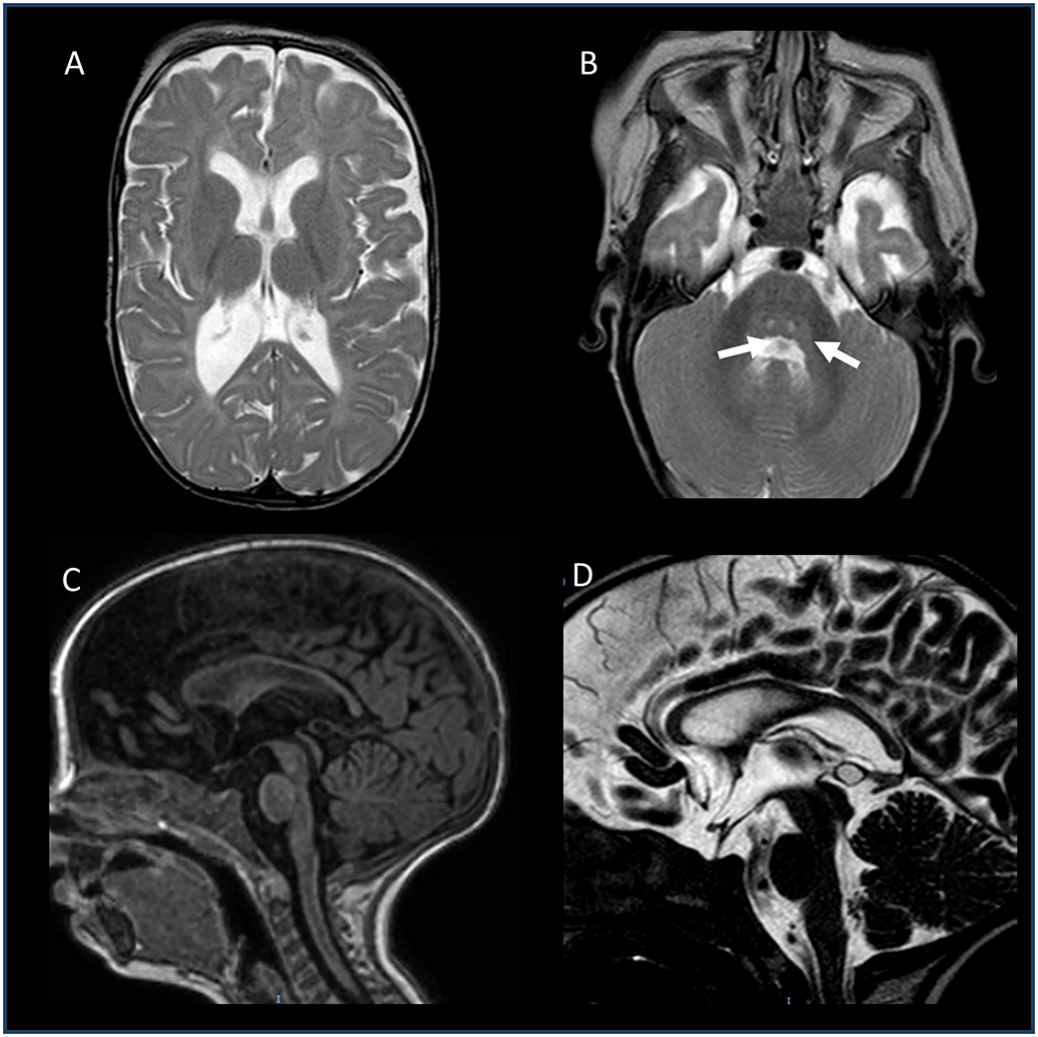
Brain MRI of the patient, performed at the age of 8 months. (A,B) Axial T2-weighted images: enlarged posterior horn of lateral ventricles with periventricular white matter thinning (A) and central tegmental tracts hyperintensity (white arrows). (C) Sagittal T1-weighted image and (D) Sagittal T2-DRIVE: Thinning of the corpus callosum and mild pons hypoplasia. No abnormalities recorded in the hypothalamic-pituitary axis.
